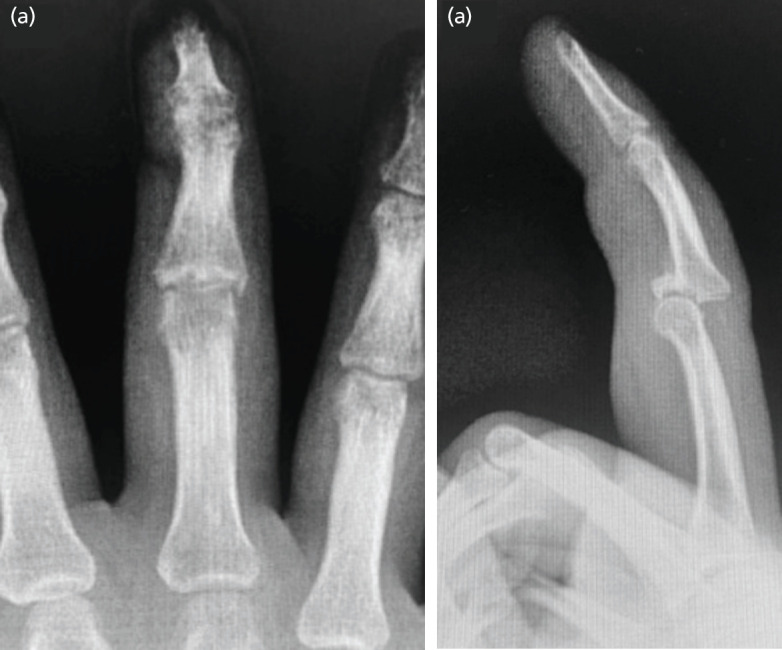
(a) Middle finger AP and (b) lateral radiographs demonstrating a comminuted volar lip fracture involving approximately 40% of the articular surface of the middle phalanx with dorsal dislocation of the PIPJ.
